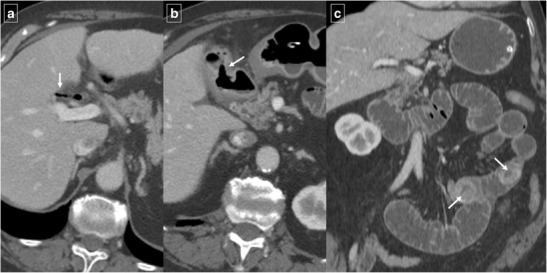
Right upper quadrant pain and gallstone ileus in a 39-year-old woman. Axial contrast-enhanced CT (a and b) shows air in the bile duct (arrow in a), a thick-walled gallbladder, and a fistula to the duodenum (arrow in b). Reformatted coronal contrast-enhanced CT image demonstrates two gallstones (arrows) within the proximal ileum with proximal jejunal dilation
